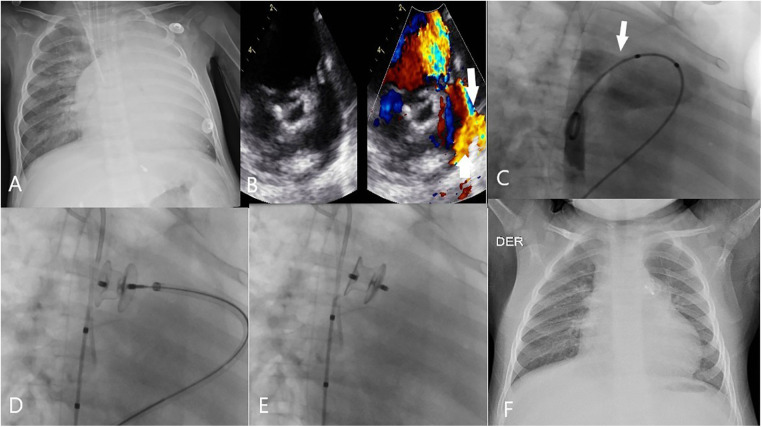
Percutaneous closure of patent anterograde ductus arteriosus with a KONAR-MF™ 12/10. (A) A chest X-ray prior to percutaneous closure of PDA. (B) TTE where we visualize the PDA (white arrow). (C) Aortography in LAO 90°/0° projection showing tubular patent ductus arteriosus (white arrow). (D) Deploying the device at the aortic level. (E) The device released. (F) A chest X-ray 6 months after percutaneous closure of the ductus arteriosus.
